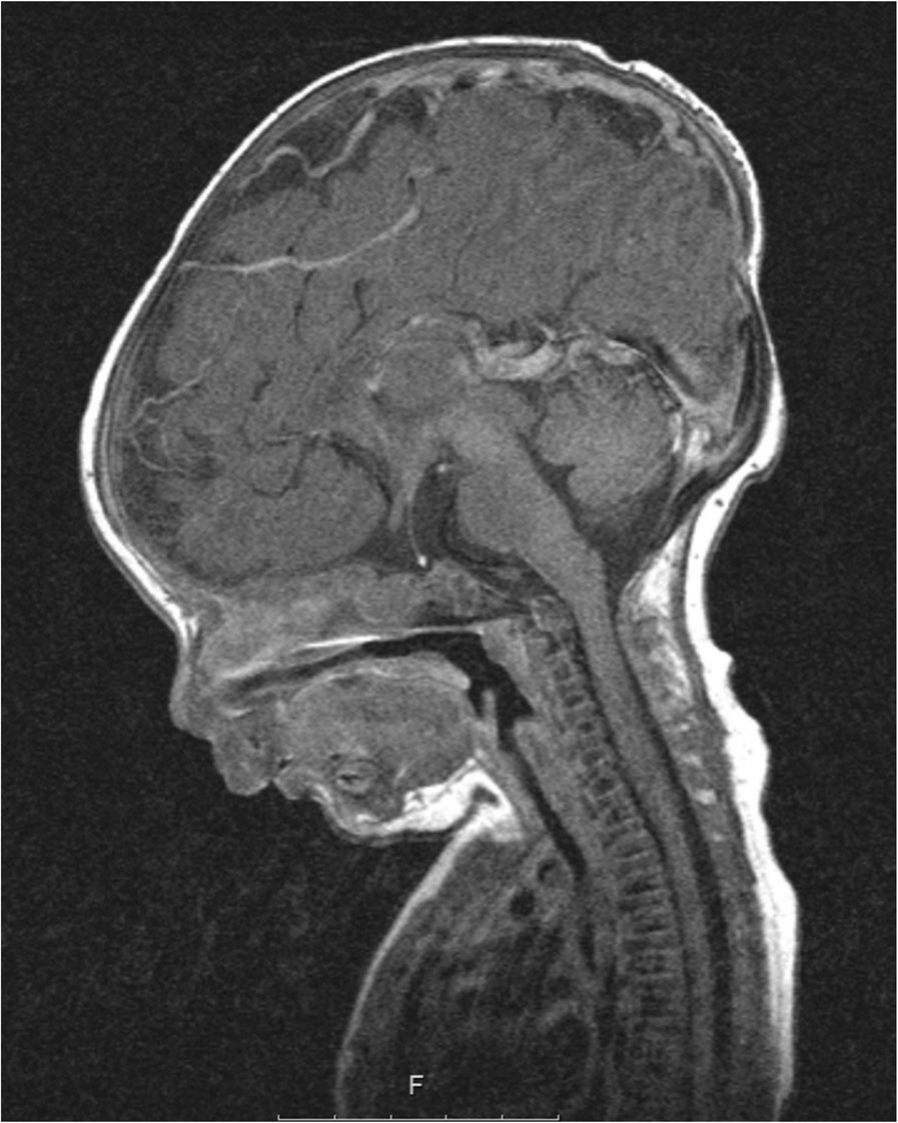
T1 sagittal MRI. Adenohypophysis is morphologically not definable, therefore, aplasia is suspected. The neurohypophysis presents as punctuate signal enhancement midsize of the hypophysis stem
